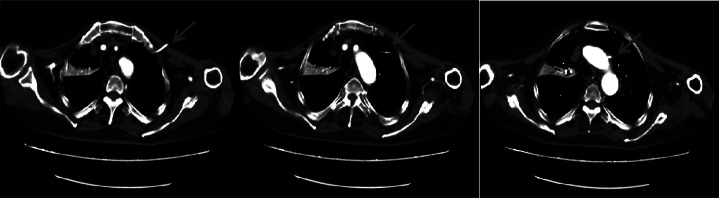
Chest CT scan showing accidental cannulation of the left subclavian central venous catheter into the aortic arch; the arrow indicates the aortic puncture site.
